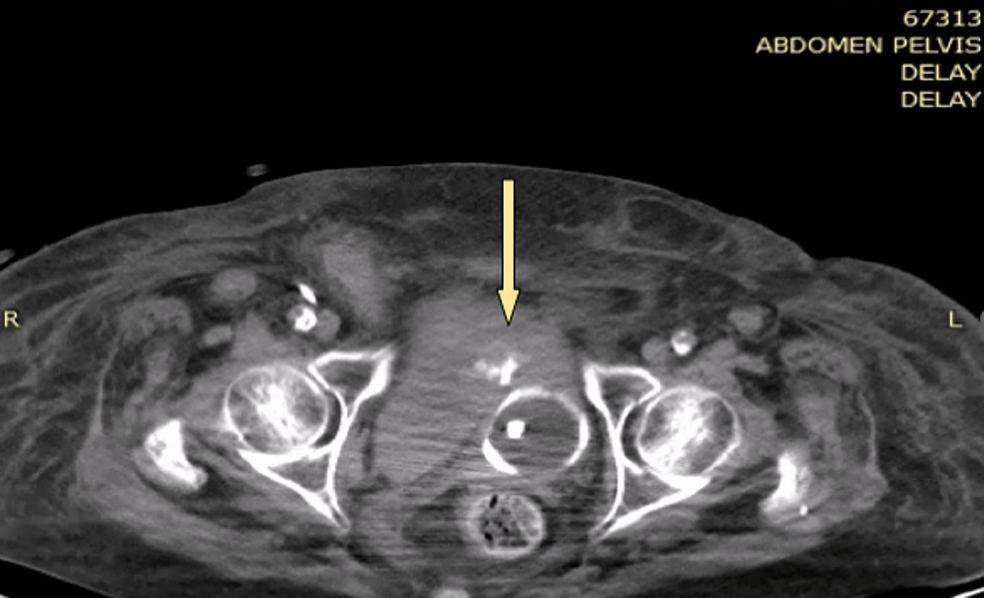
CT cystography showing contrast extravasation into the prevesical space (axial) CT: computed tomography
